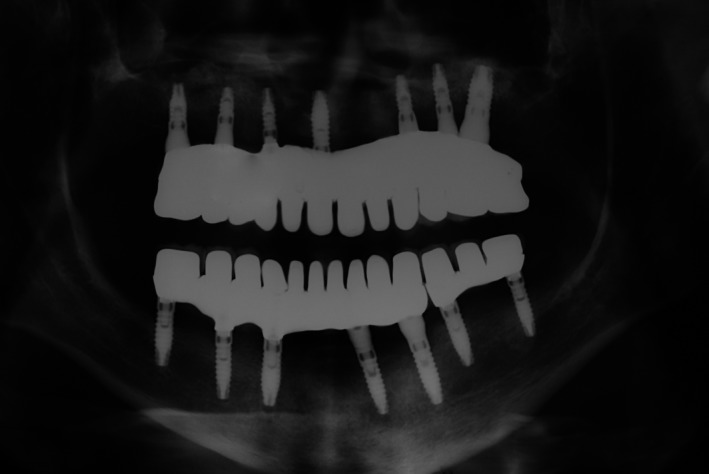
Post‐treatment radiograph.
